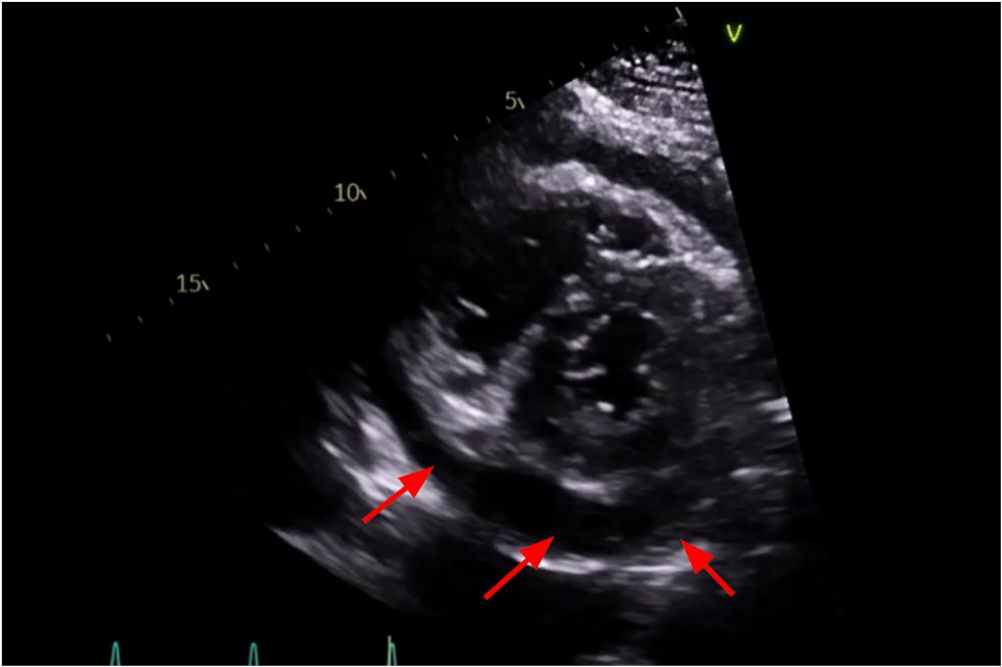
Initial transthoracic echocardiogram. Parasternal short-axis view at the mid-papillary level highlights a pericardial effusion. The anechoic (red arrows) region surrounding the heart, seen most prominently posteriorly, represents fluid accumulation within the pericardial sac. The effusion appears circumferential, with no evidence of tamponade physiology.
